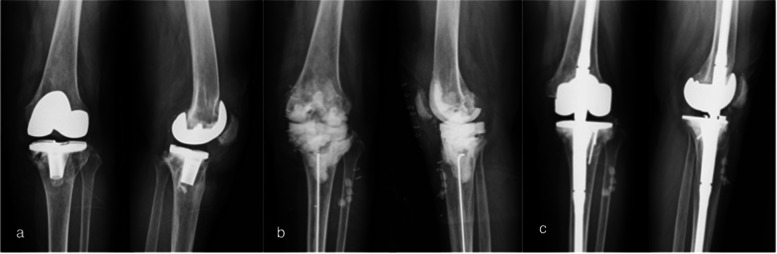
Radiographs of a 50-year-old female showing a) septic status of a left total knee arthroplasty b) 1 months after the first-stage operation with large tibia defect and reconstructed with antibiotics cement prosthesis and c) 2 years after revision with reconstruction of the tibia plateau defect restored by a femoral head allograft in structural type
